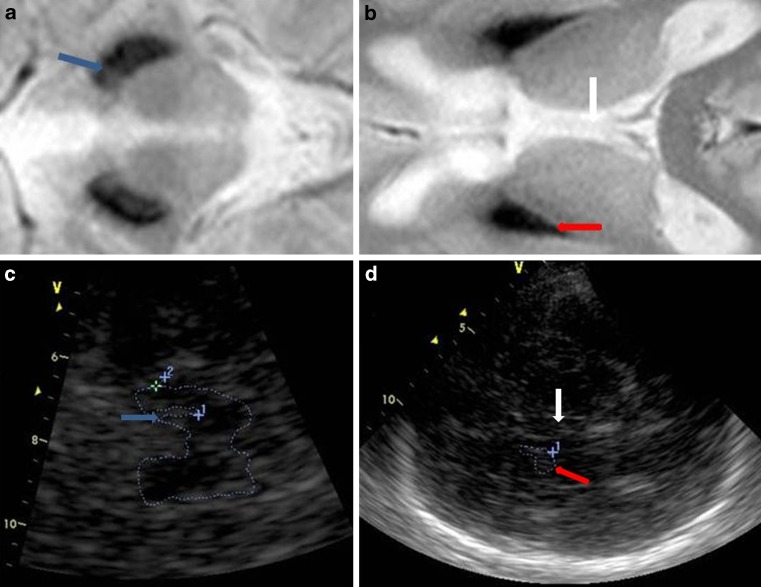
Imaging results from Patient 4. a and b, T2* MRI images of the substantia nigra (a) and globus pallidus (b). The third ventricle is indicated by a *vertical arrow*. c and d, Corresponding transcranial sonography images. The substantia nigra is indicated by an *blue arrow* (c). The area of hyperechogenicity is outlined in the medial lenticular nucleus, corresponding to the anatomical globus pallidus (d) – *red arrow*
